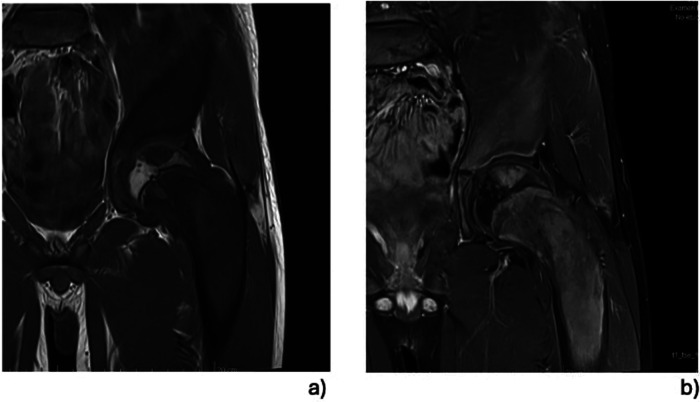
MRI focusing on left proximal femur lesions: the focus of the FD of the proximal epiphysis and the significant involvement of the femoral neck can be seen precisely. The examination did not demonstrate transphyseal diffusion of the FD, and the lesions seemed not to originate from the epiphyseal growth plate. All the lesions were characterised by a low signal intensity on T1-weighted images (a), but these were significantly better in the T1-weighted images enhanced with gadolinium (b).
